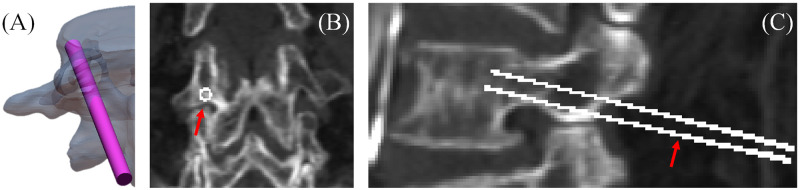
(A) Cylinder placed along the CBT path. (B) coronal and (C) sagittal CT images of a lumbar spine labeled with sampling points redefined from the coordinates of postdisplacement cylindrical mesh model vertexes.
